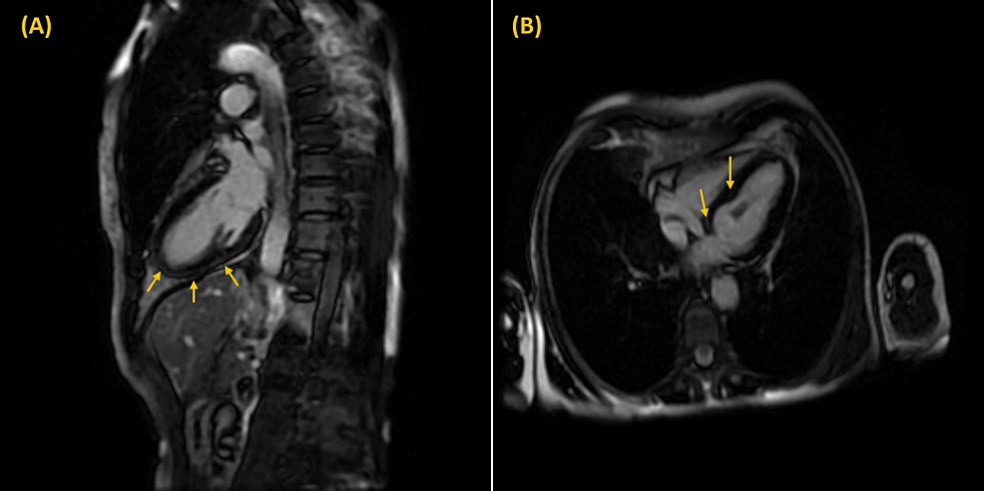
Cardiac magnetic resonance imaging Arrows in (A) indicate subtle delayed enhancement at the subepicardial region at the inferior wall extending from base to apex; arrows in (B) indicate subtle delayed enhancement at the subepicardial to midmyocardium region at the septum at the base, mid-level, and apex.
